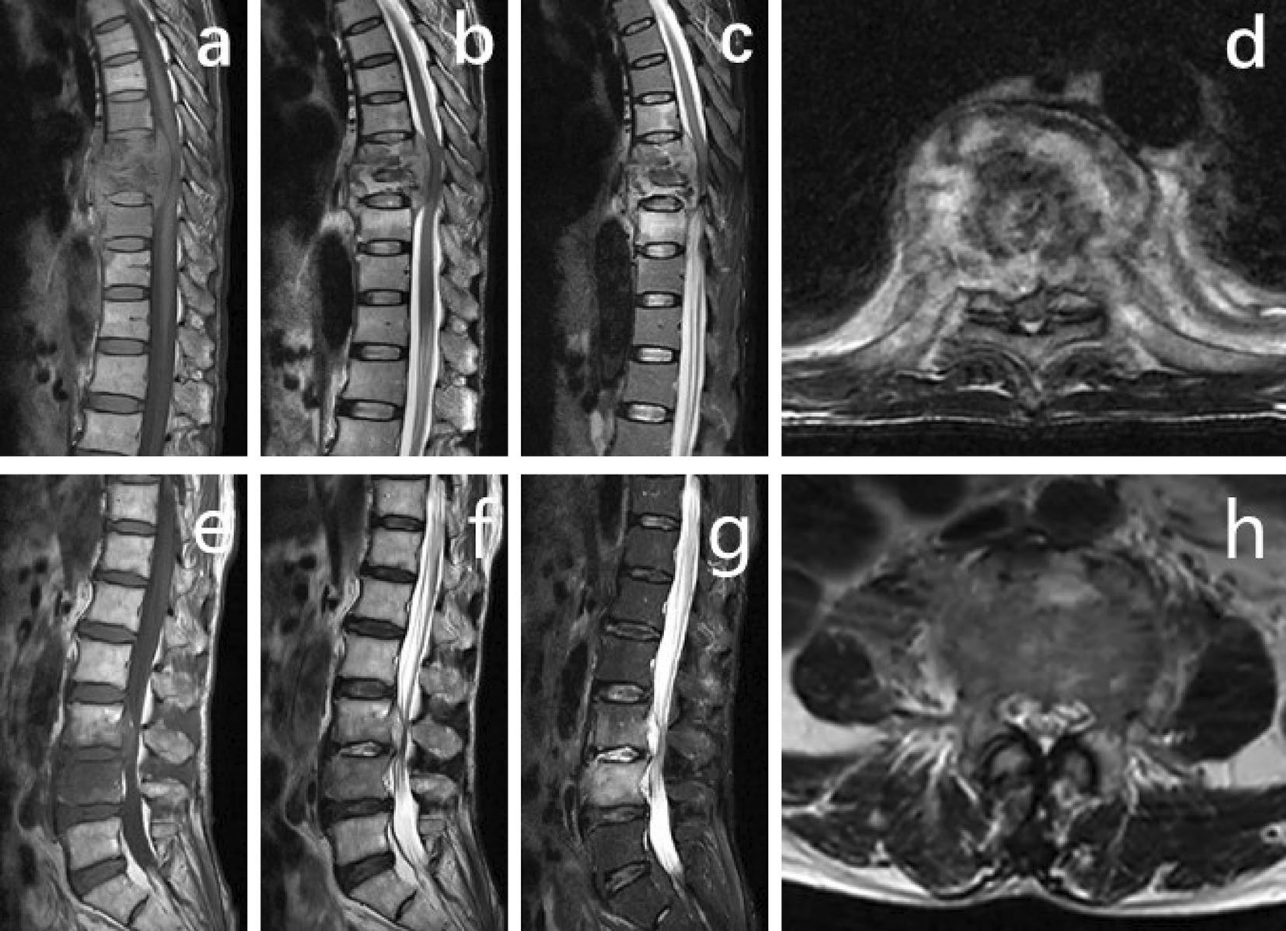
MRI scan images of tuberculous spondylitis and pyogenic spondylitis. (a–d) MRI scan images of tuberculous spondylitis. (e–h) MRI scan images of pyogenic spondylitis. In the abscesses, tuberculous spondylitis is more likely than pyogenic spondylitis to involve multiple segments with paravertebral spread.
